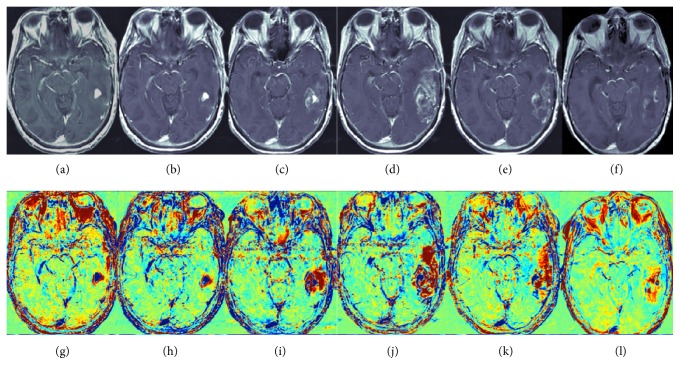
Contrast-enhanced T1-weighted images (a–f) and the calculated TRAM postchemoradiation (g–l) (images were acquired 0.7, 2.5, 4, 6, 7, and 8 months postchemoradiation). Temporary enlargement of enhancing lesion (c-d) is shown; as it can be seen, the red volume growth rate was prevalent above the blue volume (i-j), favoring pseudoprogression over progression. Pseudoprogression was later confirmed by the decrease in all volumes 7 and 8 months postchemoradiation (e-f, k-l). Modified from [110] with permission.
